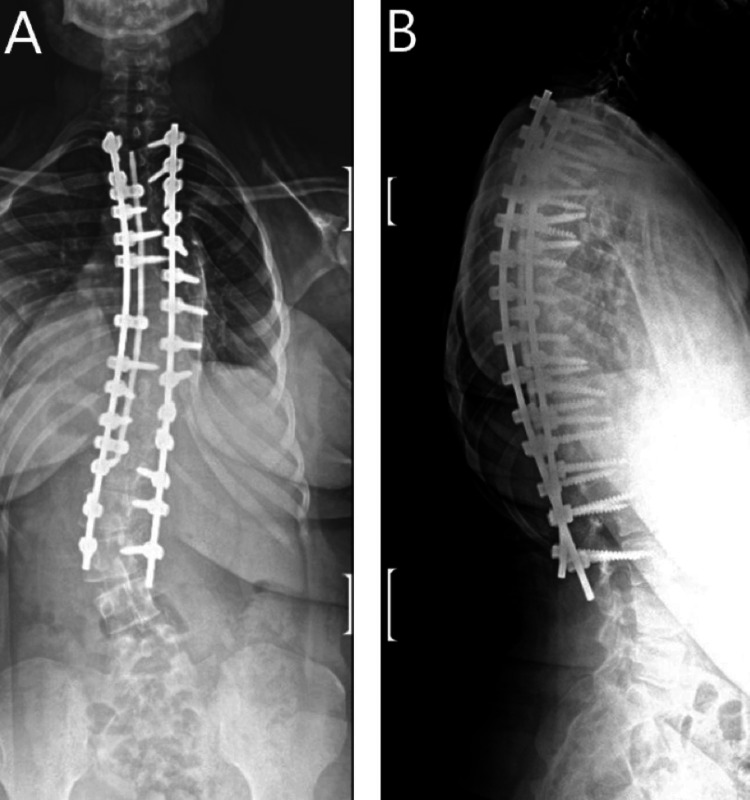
Anteroposterior (A) and lateral (B) post-operative X-ray showing T2 - L3 fusion.
